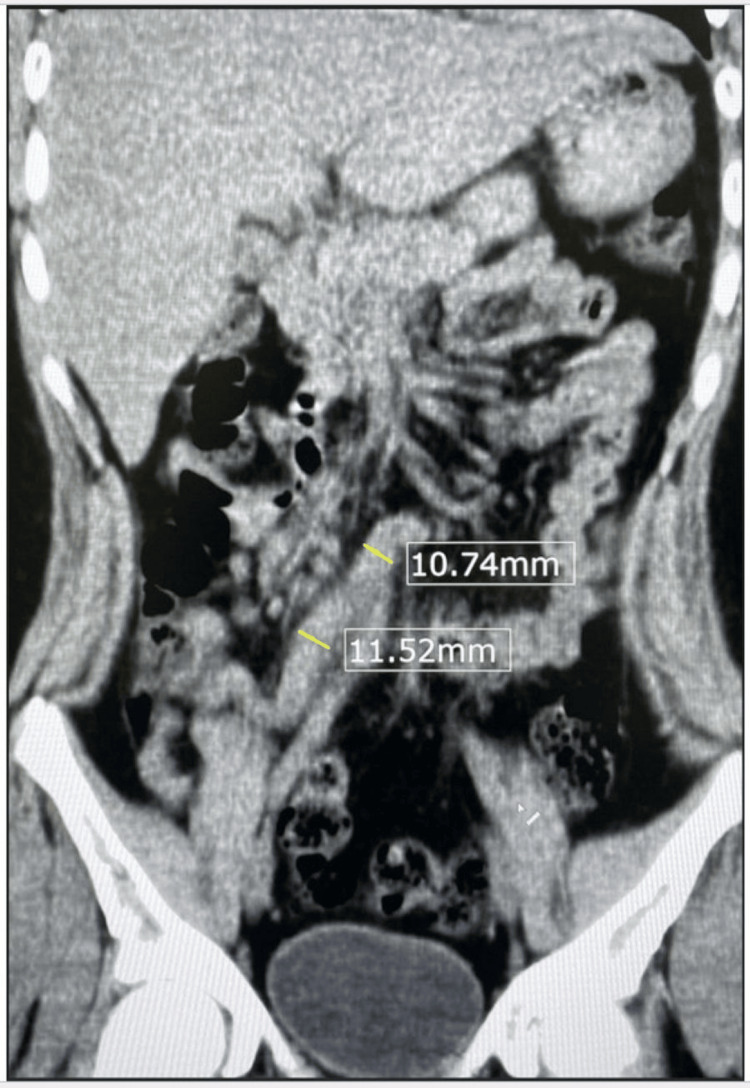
CT Abdomen findings consistent with acute appendicitis
